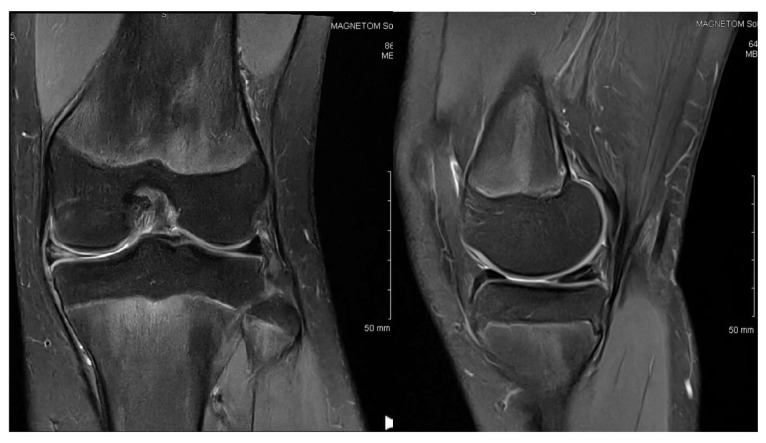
Patient S.C.S. (13 years old). Six-month postoperative MRI of the left knee following arthroscopic treatment for a medial discoid meniscus (left—coronal T2 view; right—sagittal T2 view). The coronal view showed normalization of the meniscal contour compared to the preoperative images, with resolution of the meniscal thickening. The sagittal view demonstrated no signs of retearing or signal changes in the posterior horn. No joint effusion was observed. These imaging findings were consistent with her clinical improvement and successful return to activity.
